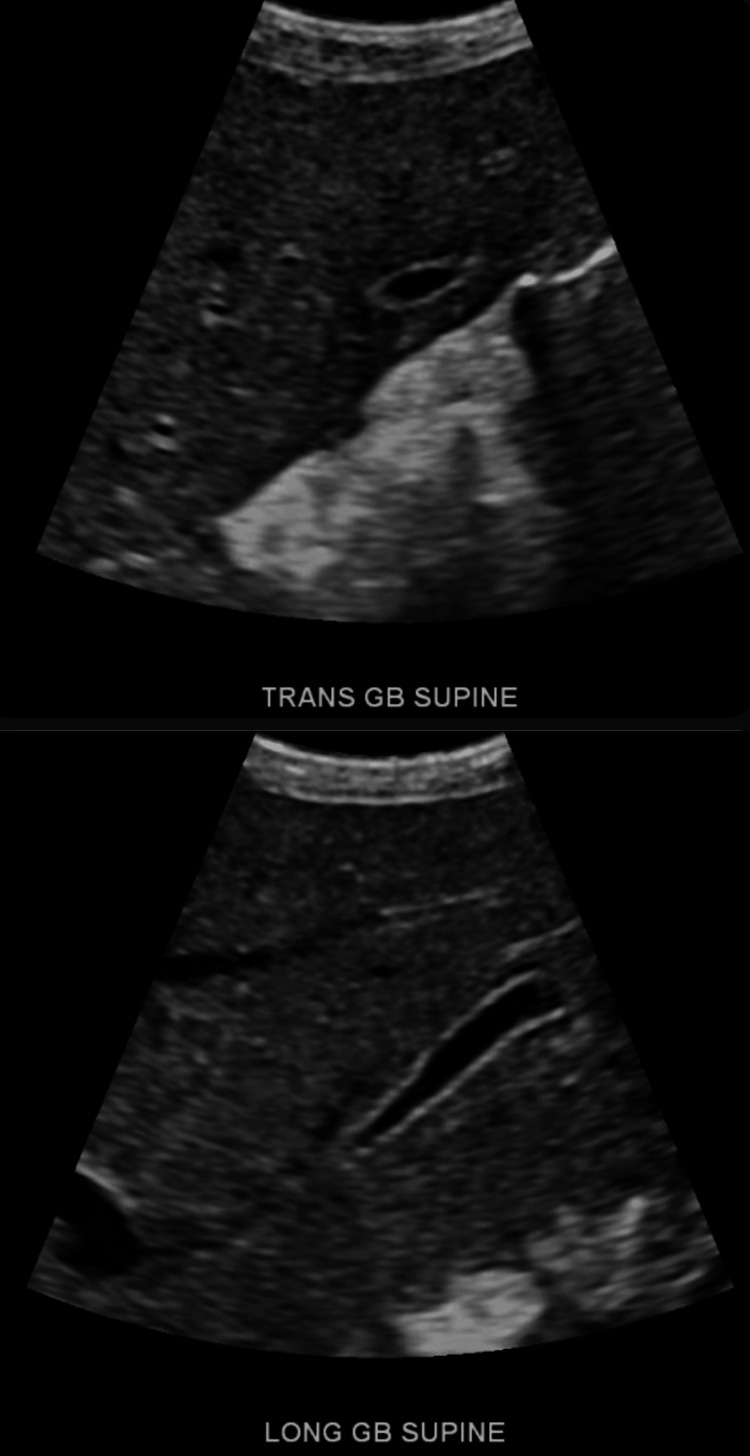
Supine transverse and longitudinal ultrasound views of the gallbladder showing a small, shrunken, and decompressed but not collapsed gallbladder, with no evidence of gallstones, biliary sludge, or biliary obstruction
